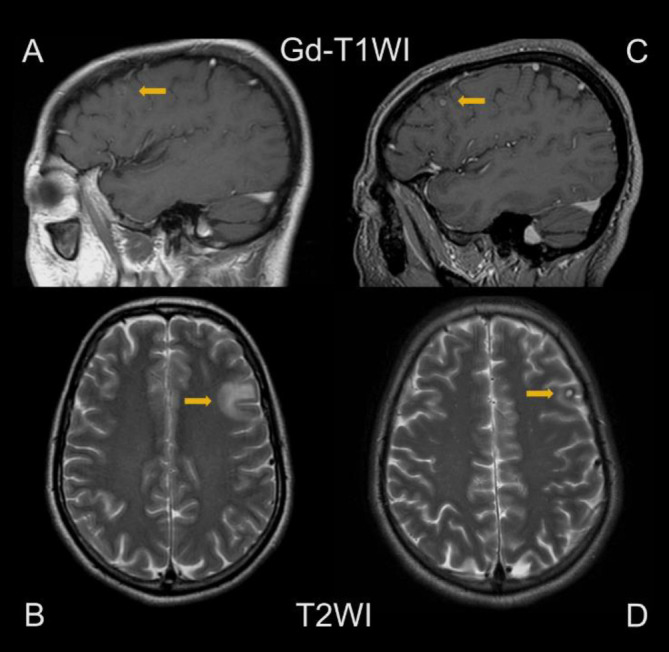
Brain MRI 1 month after the 1st radioactive iodine therapy (RAIT) (A, B) showed brain metastasis (BM) (8 mm in size) and peritumoral edema. Brain MRI 2 months after the 1st RAIT (C, D) showed shrinkage of BM and edema
